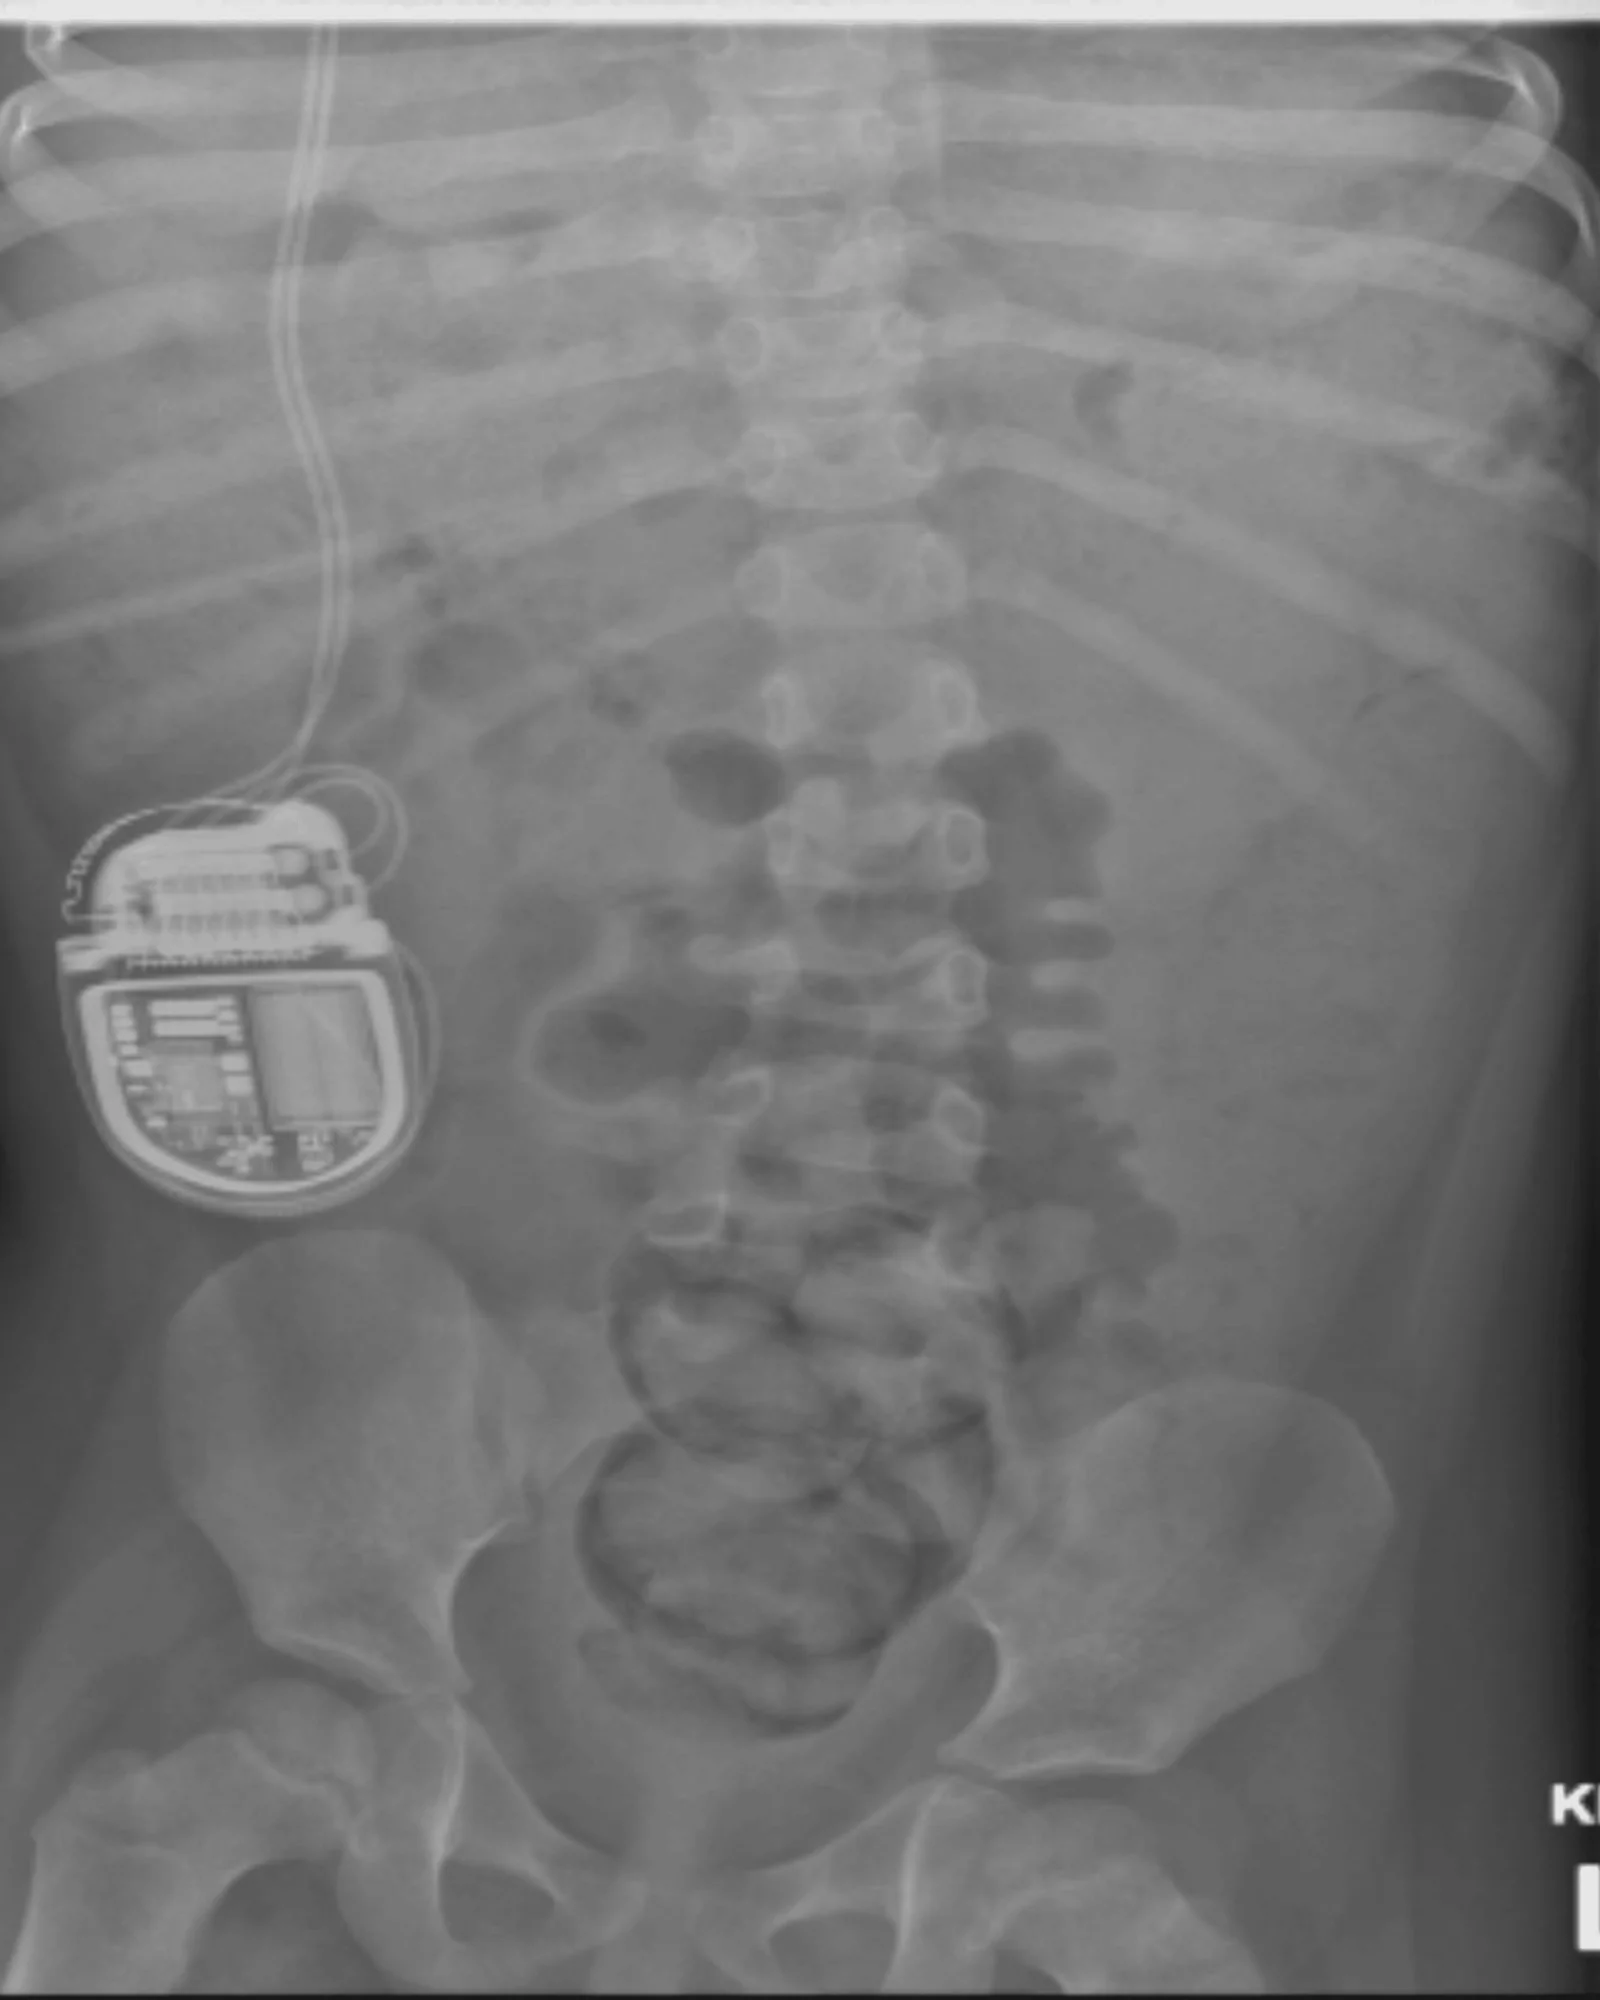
X-ray abdomen Day 5 post admission: no pneumatosis or pneumoperitoneum identified, no dilated loops; stool present in the descending colon
